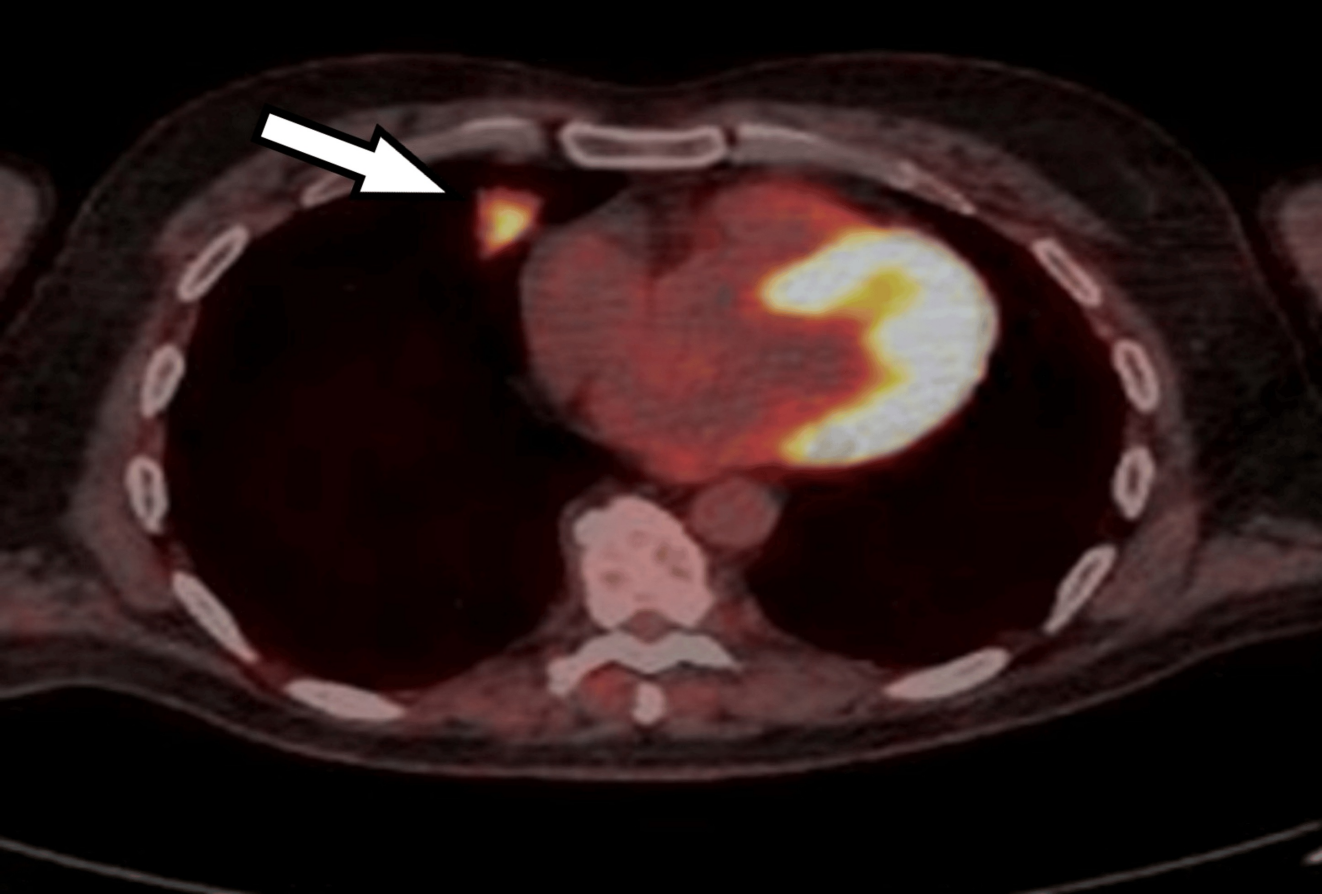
Axial PET scan of the chest demonstrating intense FDG uptake in a 31 mm soft tissue mass in the anterior aspect of the right middle lobe (white arrow) PET: positron-emission tomography; FDG: fluorodeoxyglucose
